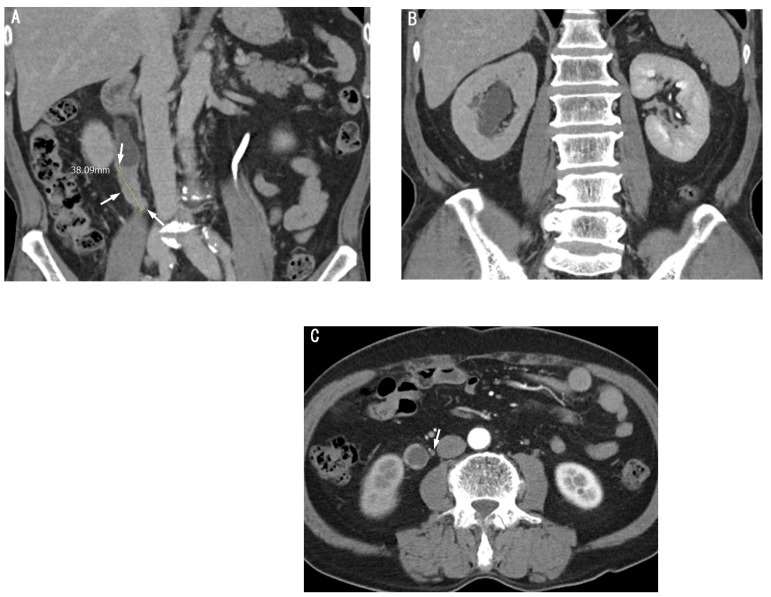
CT images of a 63-year-old male patient with ureteral urothelial carcinoma. (A) Coronal preoperative CT image shows a soft tissue mass (arrows) in the right upper ureter, causing upstream ureteral dilatation. The mass measured 3.8 cm and was pathologically confirmed as high-grade urothelial carcinoma. (B) Coronal CT image reveals marked dilatation of the renal pelvis and calyces with forniceal blunting, consistent with grade 3 hydronephrosis. (C) Axial CT image shows a small paracaval lymph node (arrow), 3 mm in short-axis diameter, confirmed as metastatic after lymph node dissection.
